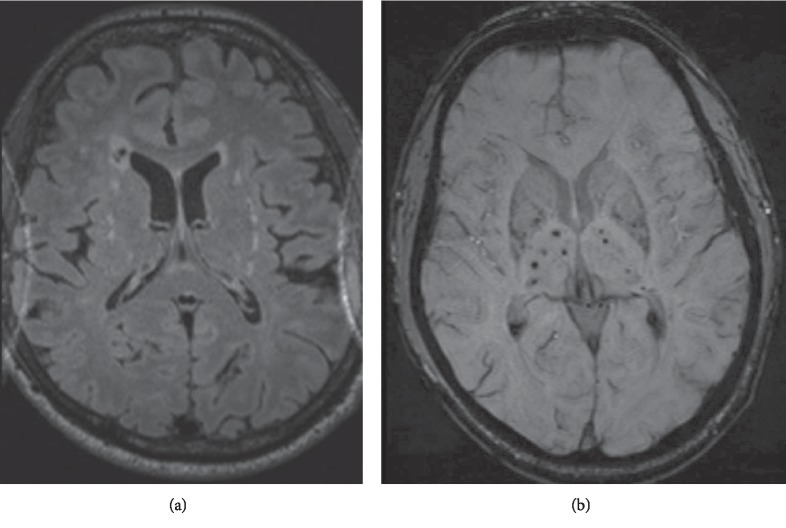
MRI brain showing abnormal FLAIR signal (a) along the bilateral external capsules, with abnormal hypo intensities in bilateral basal ganglia on T2 FFE (b).
